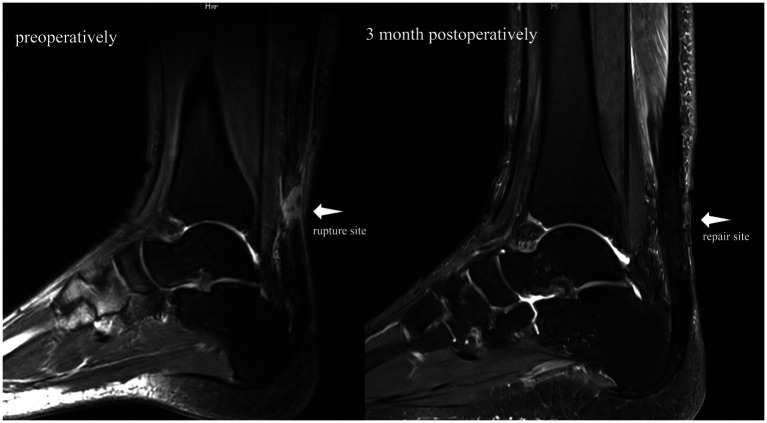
Comparison of ankle MRI images preoperatively and at 3 months postoperatively. Arrows indicate the rupture site (left) and the repair site (right), respectively.
